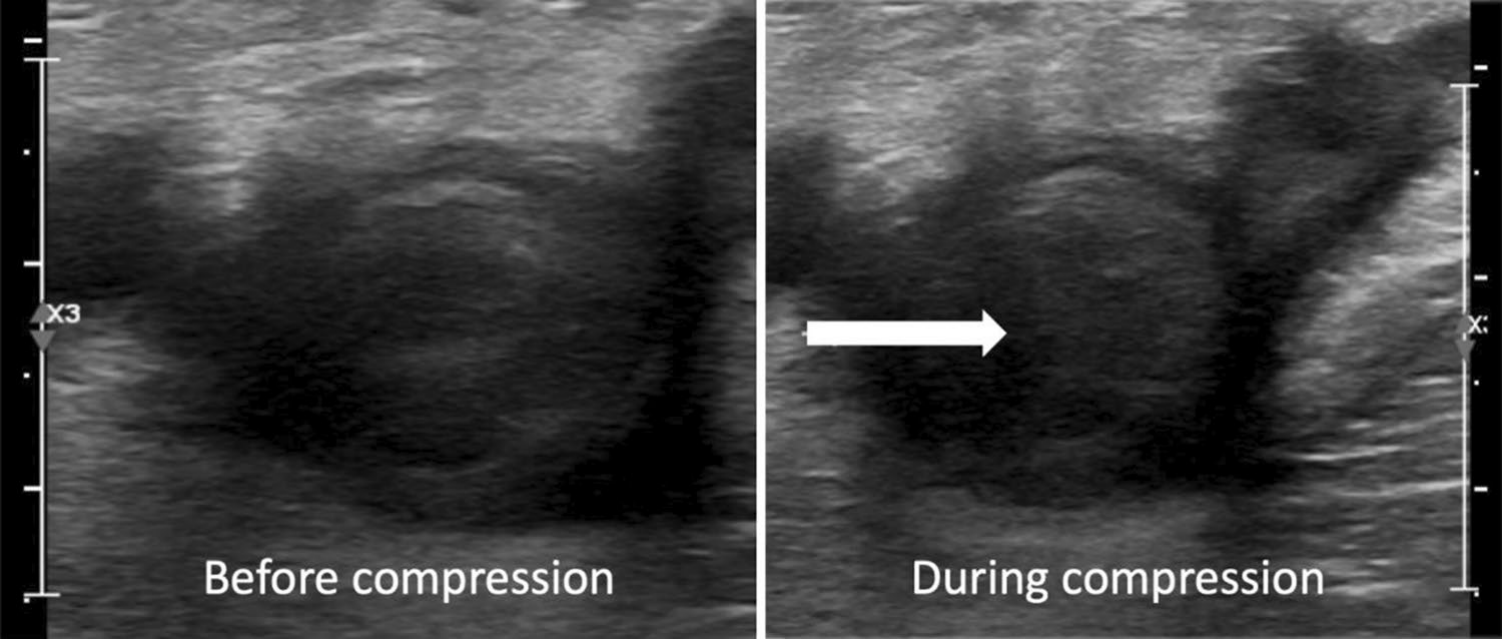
Venous compression ultrasound in a 43-year-old male COVID-19 patient, requiring mechanical ventilation on the intensive care unit (left panel: before compression, right panel: during compression). The ultrasound reveals a large, semi-occlusive venous clot in the right common iliac vein, indicated by the white arrow
